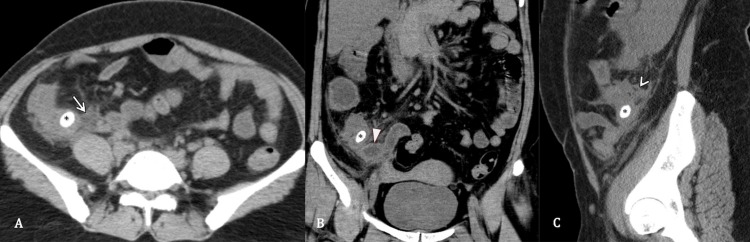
Appendicitis and appendicolith Contrast-enhanced CT abdomen, axial section (A), coronal section (B) and sagittal section (C), of a 38-year-old female patient, presented with abdominal pain and anorexia. CT shows an impacted appendicolith at the base of the appendix (asterisk) with a streak of periappendiceal fluid (arrow), dilated appendix (solid arrow head) and diffuse periappendiceal inflammation (hollow arrowhead).
